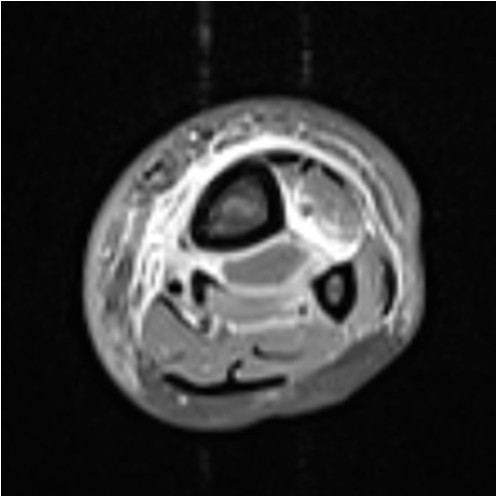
Initial magnetic resonance images of the affected tibia. The peripheral reactive effusion is explicit on liquid sensitive inversion-recovery T2-weighted axial images.
